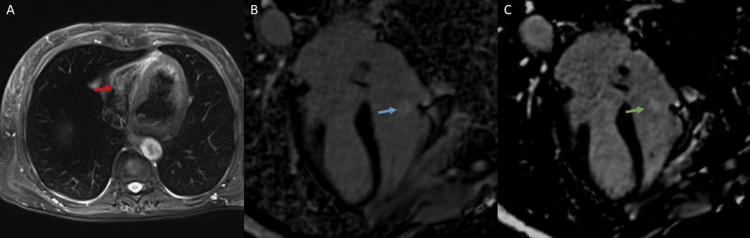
(A) Axial fat-saturated T2-weighted images demonstrate a tricuspid valve mass with hyperintense signal (red arrow). (B) Late gadolinium phase-sensitive inversion recovery sequence displays circumferential enhancement of a tricuspid valve mass (blue arrow). (C) Long inversion time (TI) late gadolinium phase-sensitive inversion recovery sequence demonstrates homogeneous enhancement of a tricuspid valve mass (green arrow).
